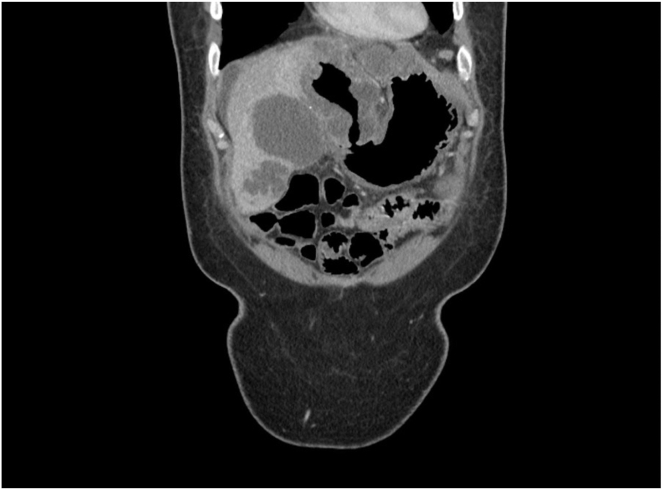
Coronal CT sections demonstrate a necrotic left hepatic mass with fistulous communication with the gastric antrum.
